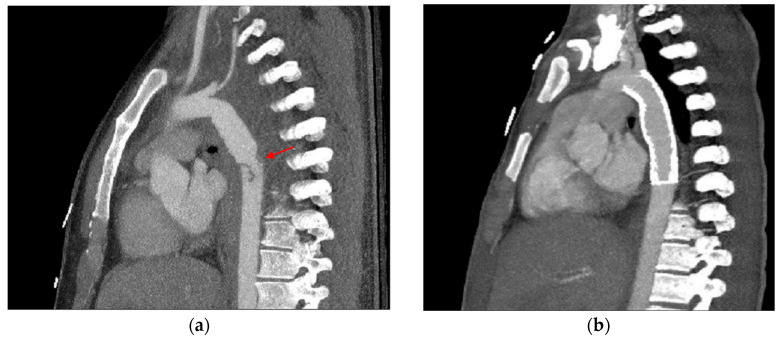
Thoracic endovascular aortic repair. (a) Sagittal CT image of a patient with grade III ATAI (red arrow). (b) Sagittal CT image following successful TEVAR.
